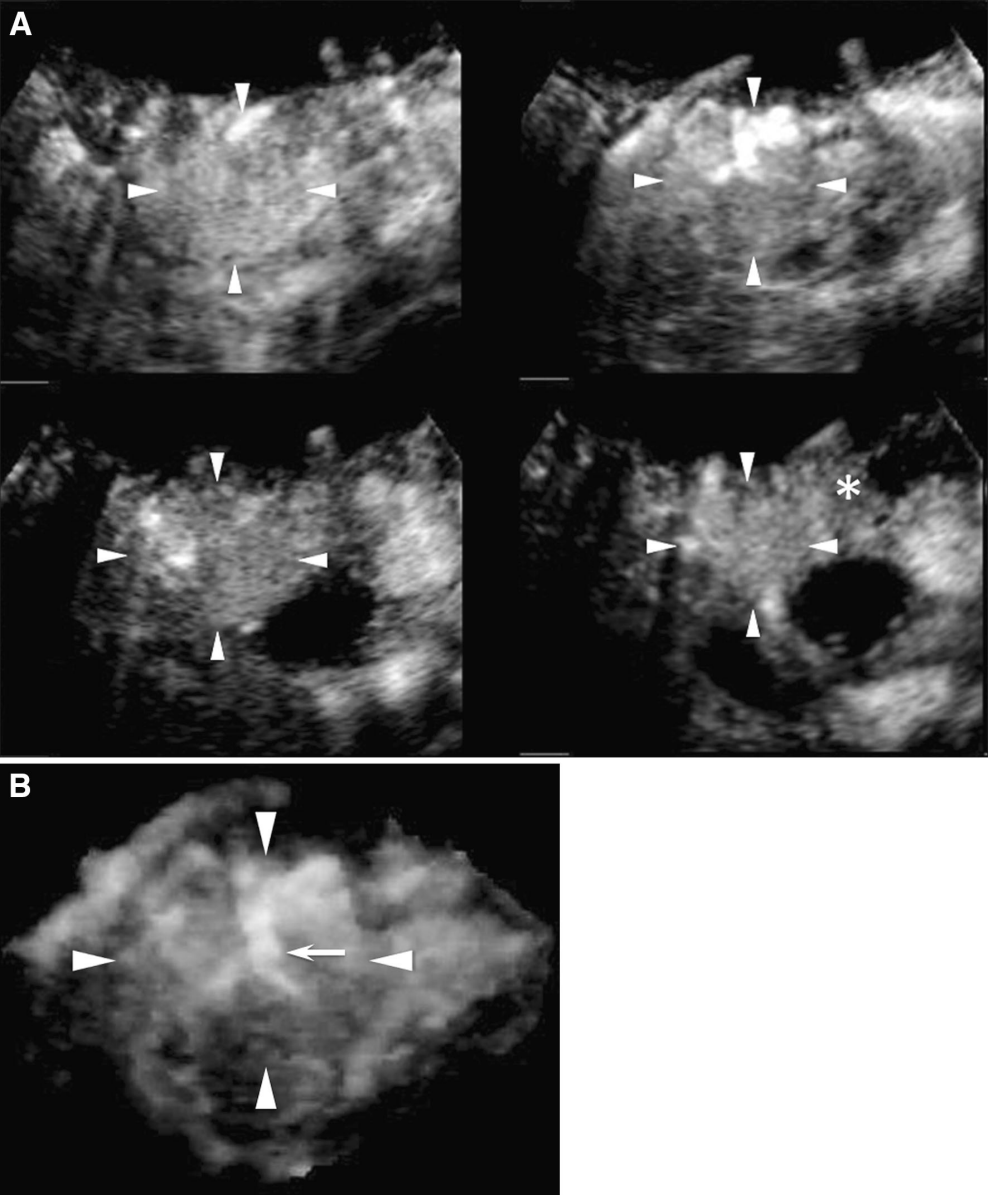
CE 3D US images acquired in the transverse plane of a 71-year-old woman with NET (maximum diameter, 38 mm) in the pancreatic head. A TUIs of CE 3D US (plane A) in the arterial phase show a hypervascular lesion (*arrowheads*) compared with the parenchyma in the pancreatic body (*asterisk*). B Sonographic angiogram in the arterial phase, rendered in the maximum intensity mode, shows a markedly dilated tortuous vessel, classified as an intratumoral and irregular vessel (*arrow*).
